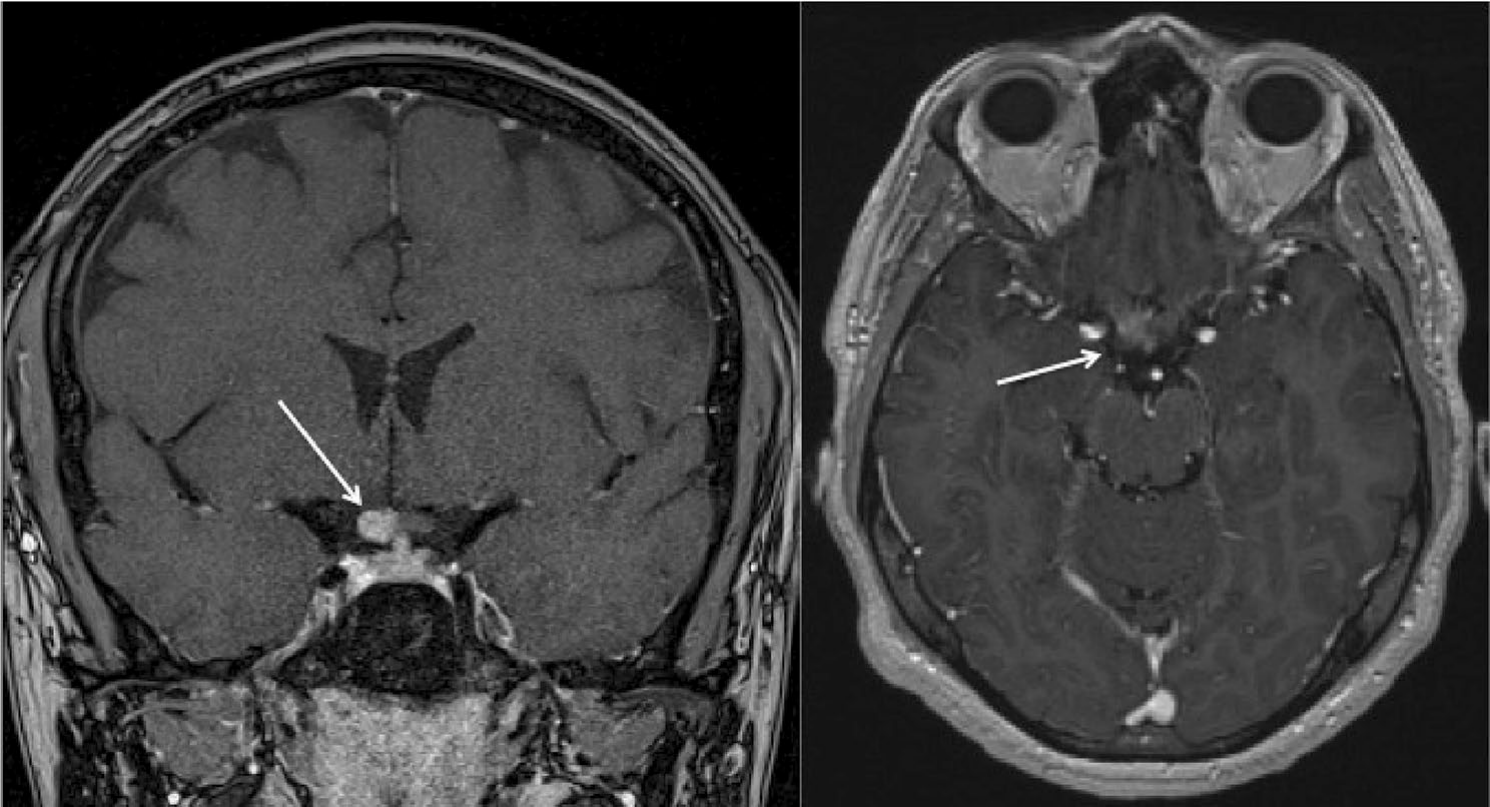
Brain MRI showing a lesion of the optic chiasm in a MOGAD patient (M2), with contrast enhancement
